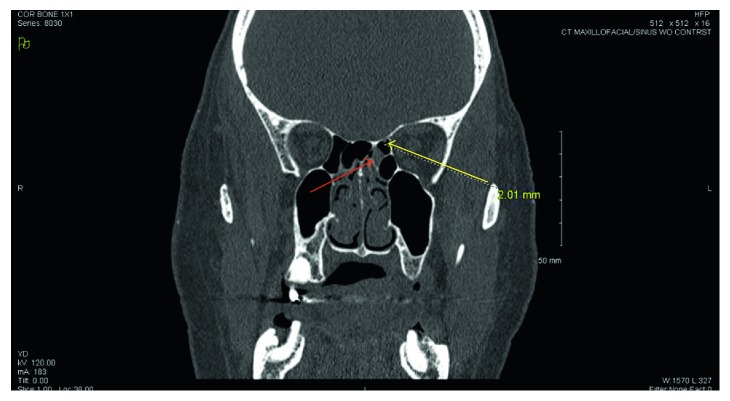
High-resolution CT showing area suspicious for a small skull base defect (yellow arrow) and adjacent opacification in the posterior ethmoid sinus (red arrow).
